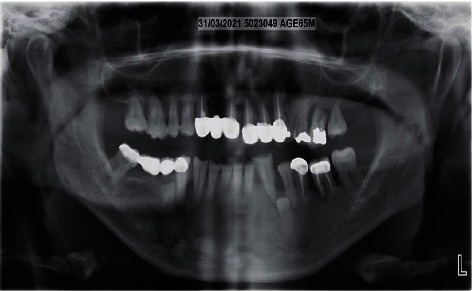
Postoperative radiograph after one year.
